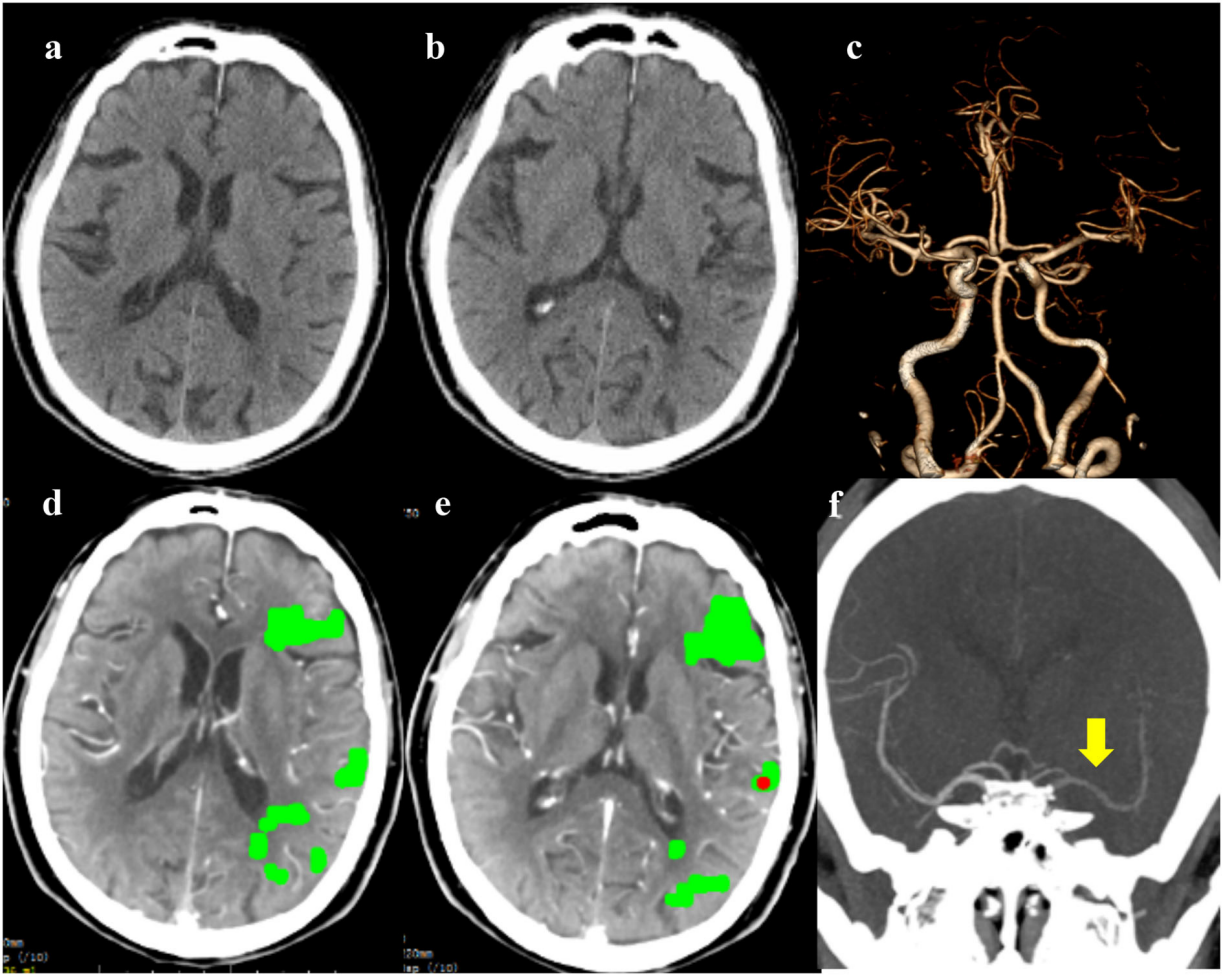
The emergency non-contrast CT (NCCT) (a,b) and cranial CT angiography (CTA) (c) did not show obvious cranial abnormalities; whole-brain CT perfusion (WB-CTP) indicated the left hemisphere core infarction (d,e); The 4D-CTA reconstruction showed delayed imaging of the left middle cerebral artery [(f), yellow arrow].
